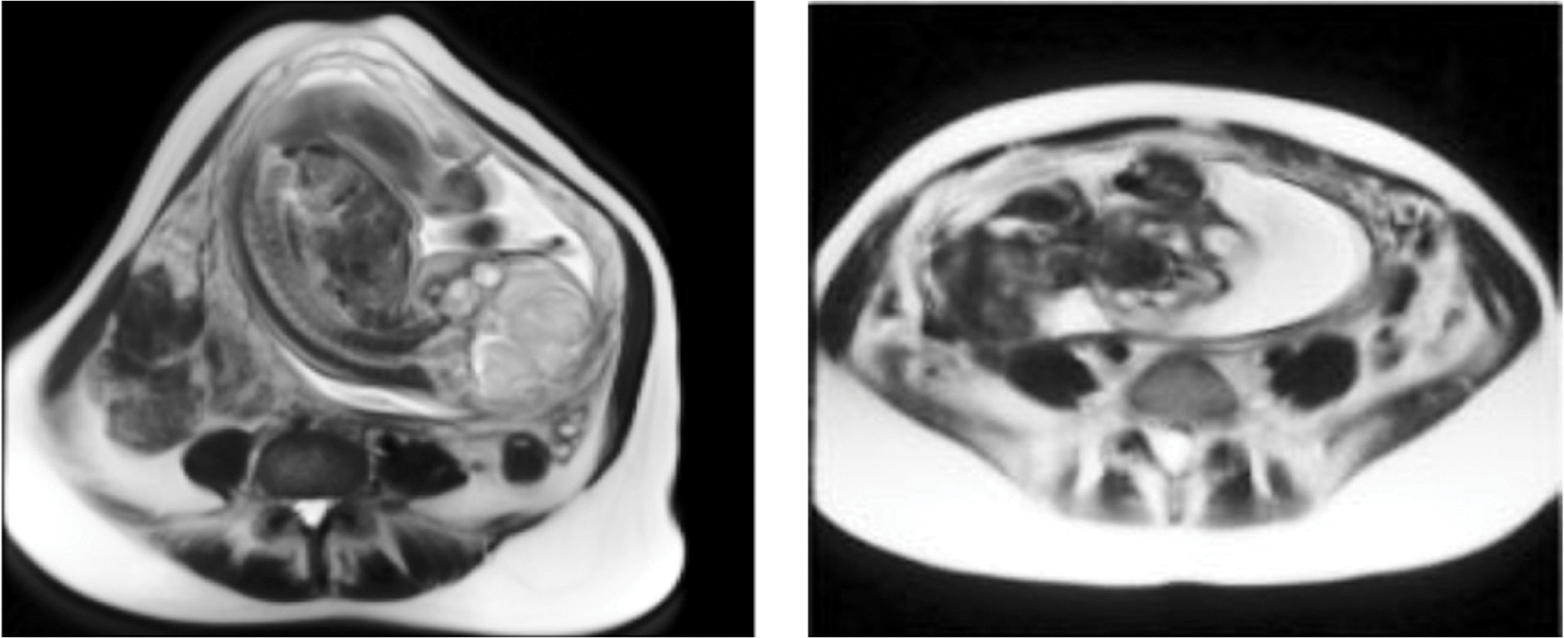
3D MRI slices of the placenta.
